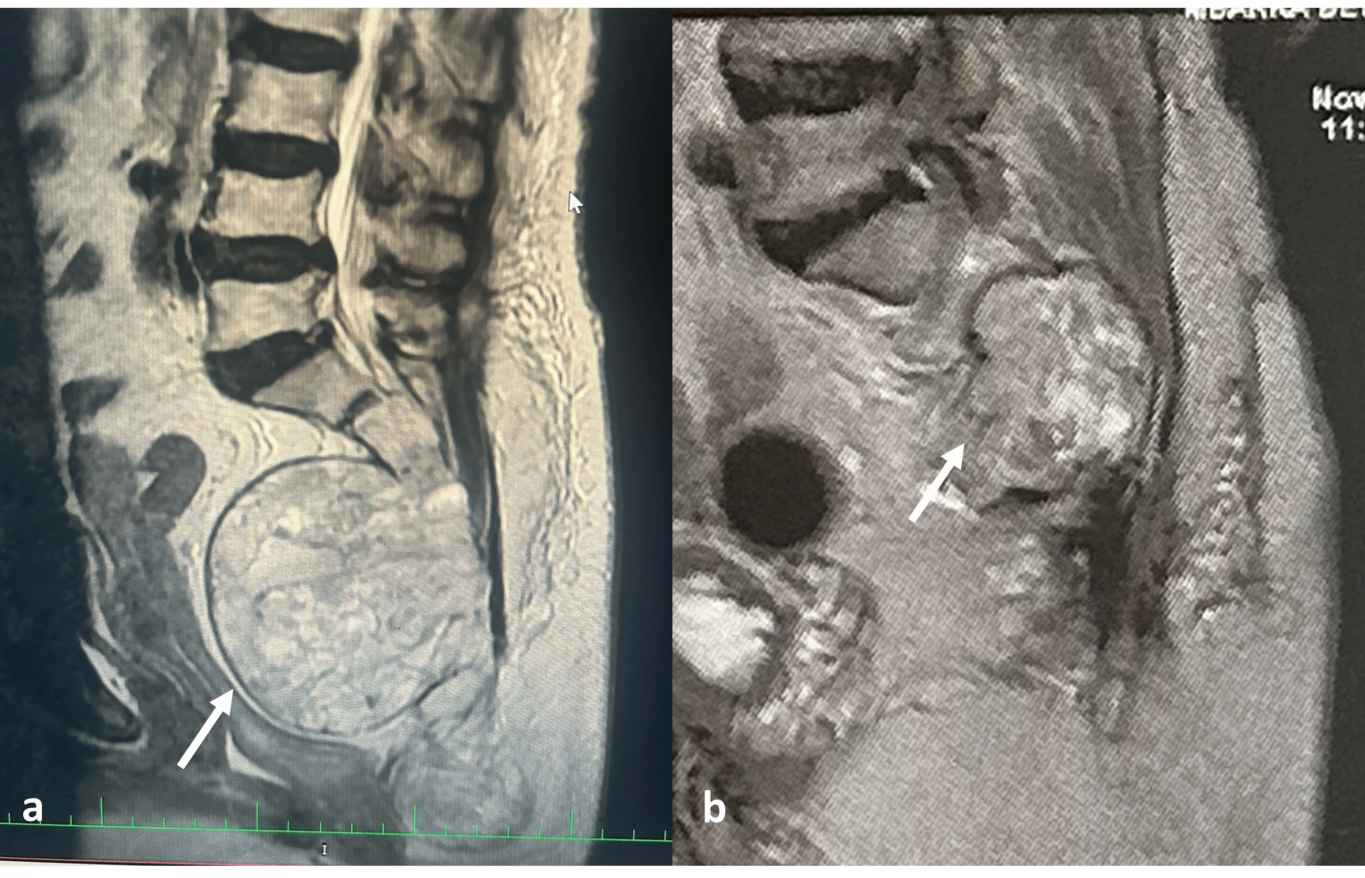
MRI findings (a) Preoperative sagittal T2-weighted MRI showing a large, well-circumscribed, hyperintense mass in the sacrococcygeal region, measuring 108 × 88 mm (arrow). The lesion causes extensive osteolytic destruction of the sacrum and coccyx, posterior compression of the cauda equina, anterior displacement of the rectum, and infiltration of adjacent soft tissues. (b) Postoperative sagittal T2-weighted MRI showing subtotal resection of the lesion (arrow) with decompression of the thecal sac and reduction of mass effect on adjacent structures.
